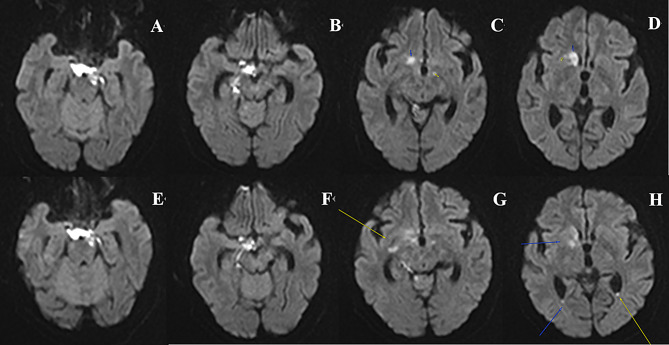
Brain MRI evolution. (A–D) Contrast-enhanced MRI (August 23): abnormal signal in the ambient and suprasellar cisterns, consistent with inflammation/early abscess formation. Right cavernous sinus widening with heterogeneous enhancement, suggestive of thrombosis. Diffusion-weighted imaging (DWI) shows hyperintense lesions in bilateral basal ganglia. (E–H) Follow-up MRI (August 26): interval increase in the number and extent of DWI hyperintense lesions within the bilateral basal ganglia, consistent with progressive cerebral infarction
